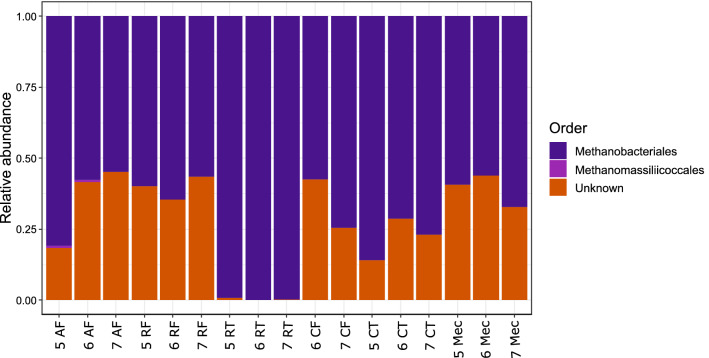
Mean relative abundance of archaeal ESVs in each sample type (GIT component and amniotic fluid) at each gestational age (5, 6 and 7 months). ESVs are clustered by colour to the level of order. ESVs with taxonomic assignments at the order level with confidence values lower than 90% are denoted as ‘Unknown’. The gDNA from caecal tissues at 5 months gestation was below the concentration threshold (0.5 ng/µL) for samples to be included in the sequencing run. Amniotic fluid (AF), ruminal fluid (RF), ruminal tissue (RT), caecal fluid (CF), caecal tissue (CT), meconium (Mec).
